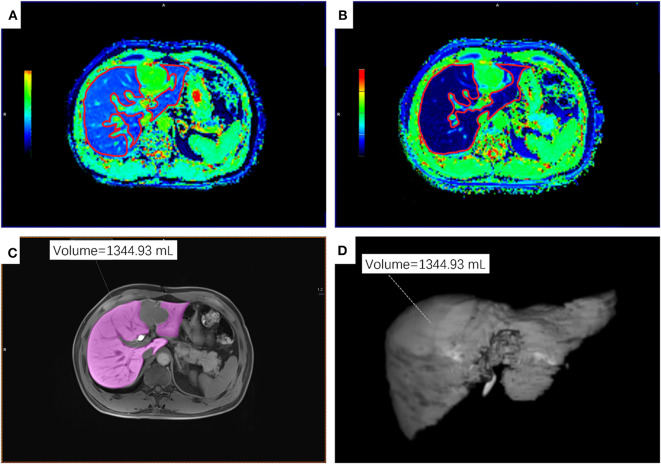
An example of ROIs on T1 maps, T1-weighted VIBE sequence and 3D volumetric images of the residual liver parenchyma. (A,B) ROIs on the T1-mapping before (A) and 20 min after (B) the administration of Gd-EOB-DTPA. (C) ROI on T1-weighted VIBE sequence. (D) 3D volumetric image. ROI, regions of interest.
